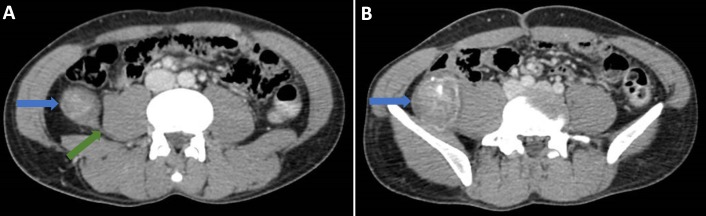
Computed tomography scan of the abdomen and pelvis in portal venous phase (A and B). A) The axial slices show a well-defined mass (blue arrows) in the right iliac fossa, which is separate from the adjacent large bowel and has a tail (green arrow) superiorly and inferiorly (see Figure 2). The mass itself shows heterogeneous enhancement with some coarse calcifications. It is located along the course of the lateral cutaneous nerve. The overall appearances are suggestive of a nerve sheath tumor. Figure 1B shows the mass with heterogeneous enhancement and calcifications (blue arrow). A soft tissue tumor, such as fibrosarcoma, was thought to be a less likely differential diagnosis at this stage.
